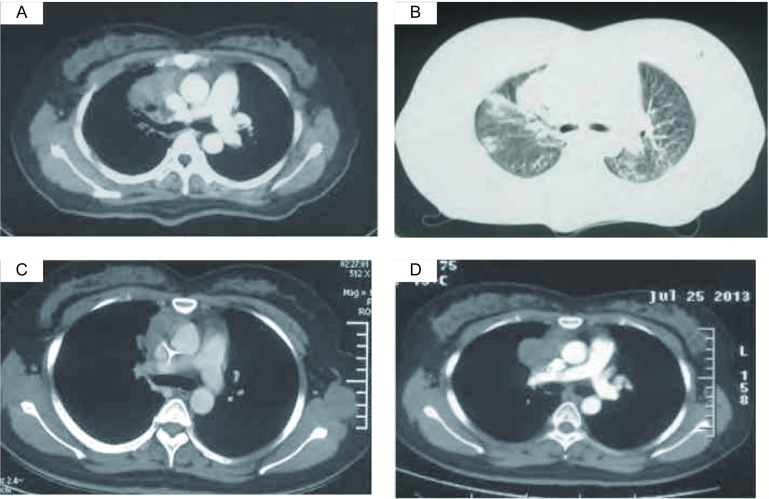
不同时间段的患者胸部CT检查。A-B：化疗前患者的胸部CT（2012-12-4），示右肺上叶纵隔旁肿物，内部可见含气支气管征，肿物周围大量磨玻璃密度影；C：第2次化疗周期结束后的胸部CT（2013-1-22），示肿物体积较化疗前略缩小；D：第5次化疗周期结束后的胸部CT（2013-7-25），示肿物体积较第2次化疗结束后明显增大。 CT scans of the chest during different times. A-B: The chest CT before chemotherapy (2012-12-4), indicating the mass in the upper lobe of the right lung. The mass was closed to the mediastinum and contained air brochogram. There were amount of ground glass density shadows around the mass; C: The chest CT scan after the second cycle of chemotherapy (2013-1-22). The volume of the mass was slightly decreased compared to the beginning; D: The chest CT scan after the fifth chemotherapy (2013-7-25). The volume of the mass was increased compared to the mass after the second chemotherapy. CT: computed tomography.
